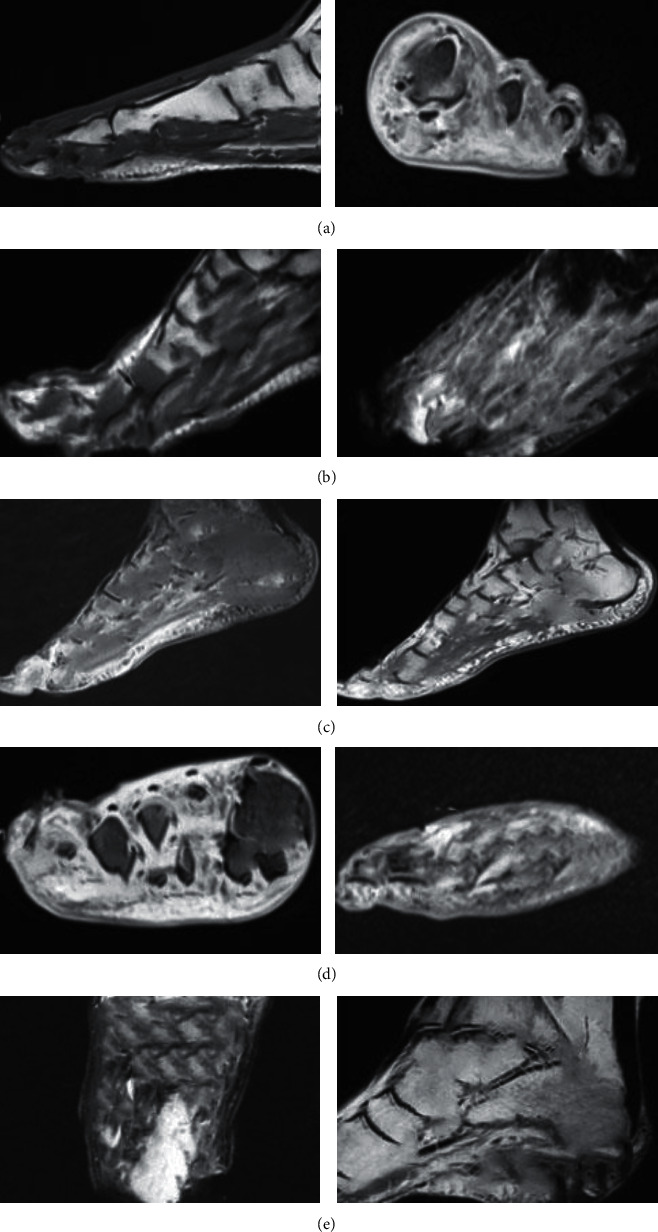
MRI images of different DF patients.
